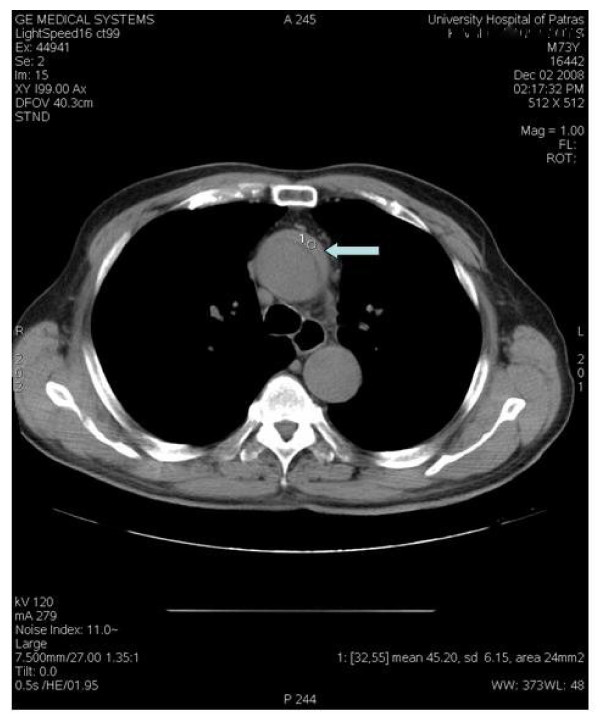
Contrast-enhanced CT reveals an intramural haematoma (IMH) of the ascending aorta located mainly in the anterolateral wall (arrow). The haematoma is appeared as a thickening of the aortic wall.
